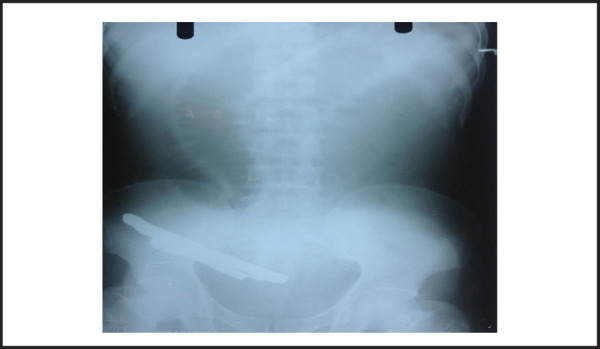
X-ray abdomen: the radiopaque shadow in the pelvic cavity and centrally multiple dilated bowel loops were noted, suggesting foreign body and likely bowel obstruction.
